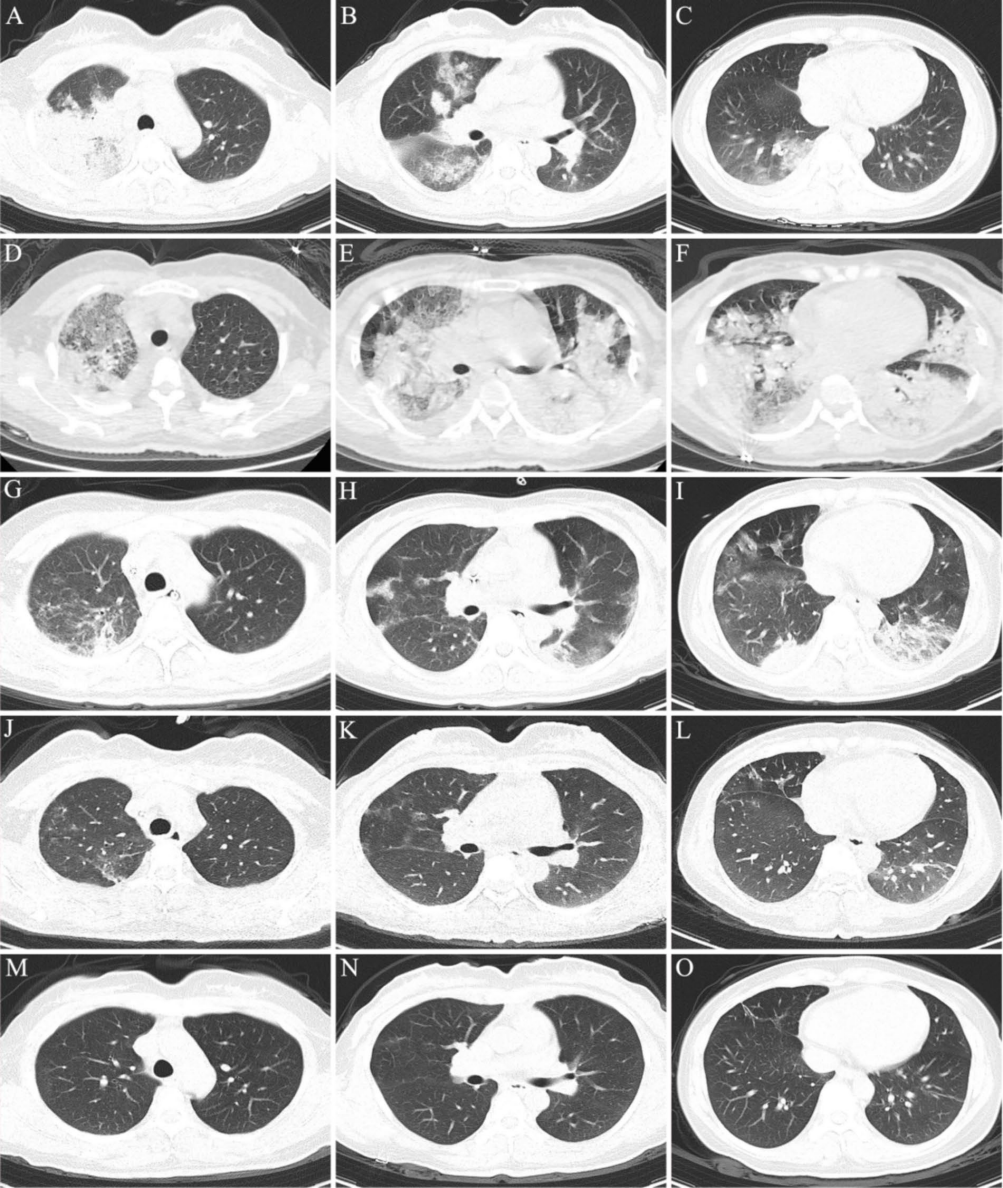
Chest CT scan of the patient during hospitalization and follow-up. (A–C) On the 1th day after admission; (D–F) on the 6th day after admission; (G–I) on the 12th day after admission; (J–L) on the day of discharge; (M–O) on the 17th day of follow-up after discharge.
